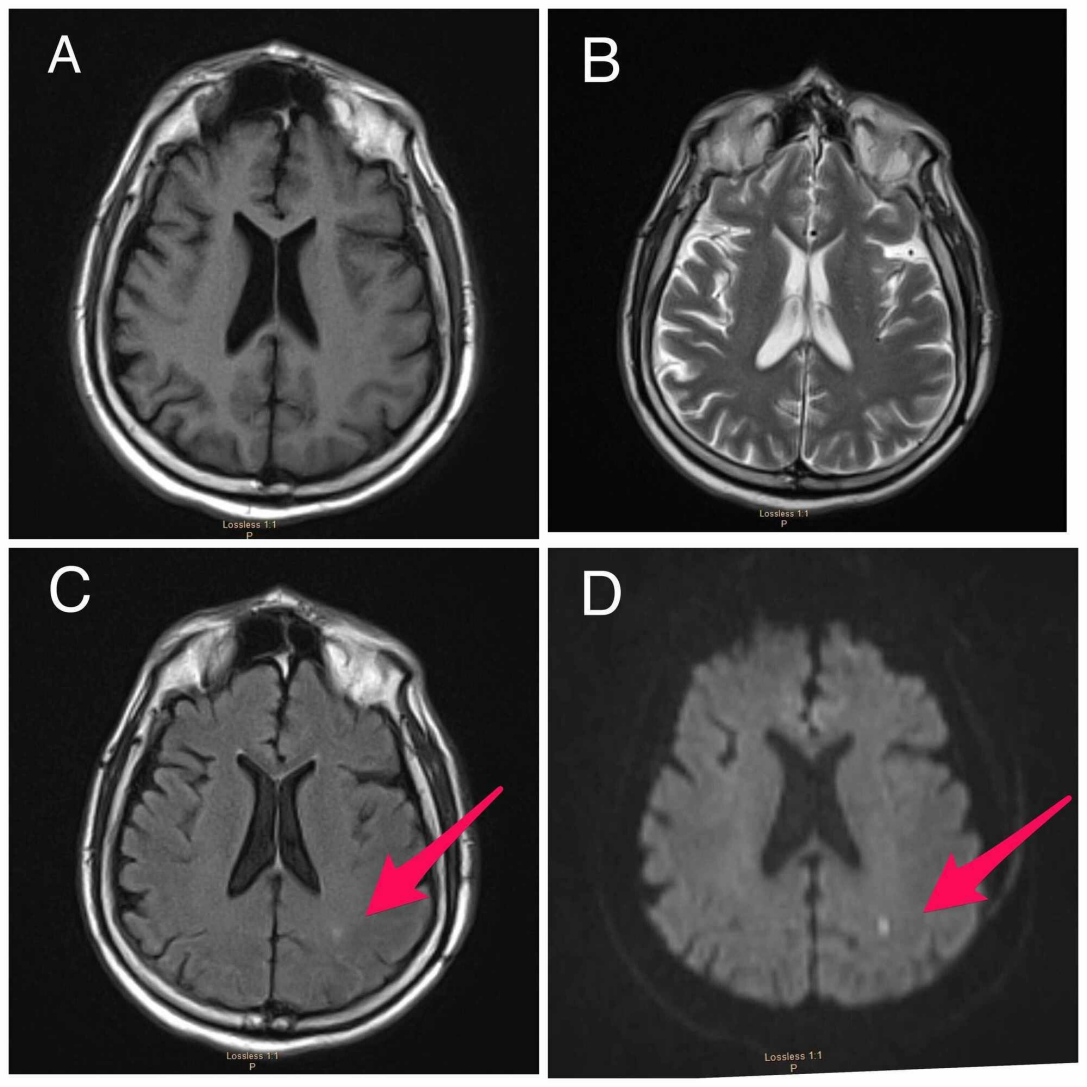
MRI of the brain. (A) Normal T1-weighted, (B) normal T2-weighted, (C) FLAIR sequence shows increased signal in the area of the acute stroke (arrow) and (D) diffusion-weighted imaging shows an area of restricted diffusion in the left parietocoritcal region (arrow). FLAIR, fluid attenuated inversion recovery.
